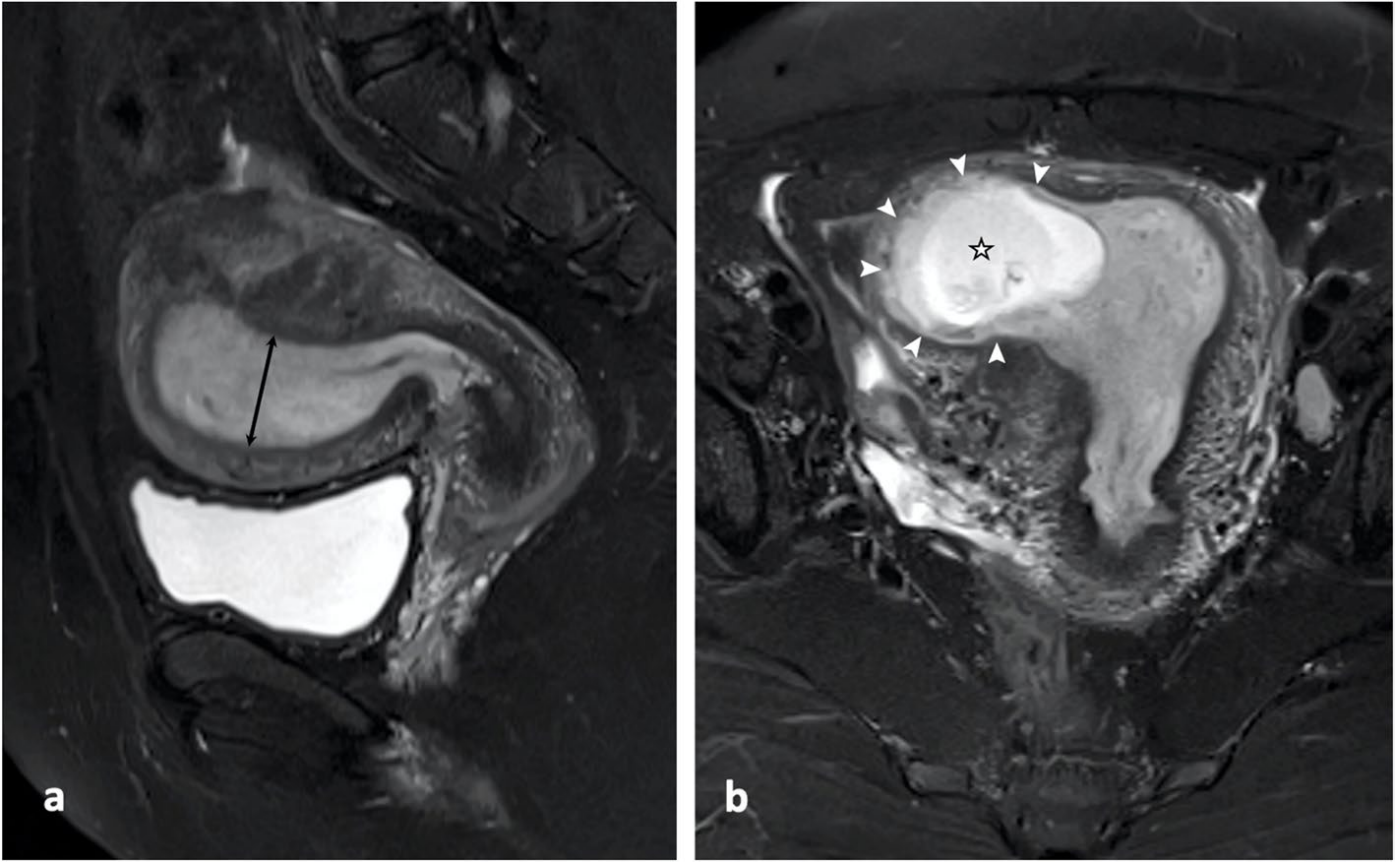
MRI in a 29-year-old patient with above-cutoff (11.5 mm) endometrial thickness. (a) Uterine sagittal fat-suppressed, T2-weighted imaging showed an endometrial thickness (double-ended arrow) of 22.6 mm. (b) Uterine coronal fat-suppressed, T2-weighted imaging showed a gestational sac (star) and continuous peri-gestational sac endometrium (arrow heads)
